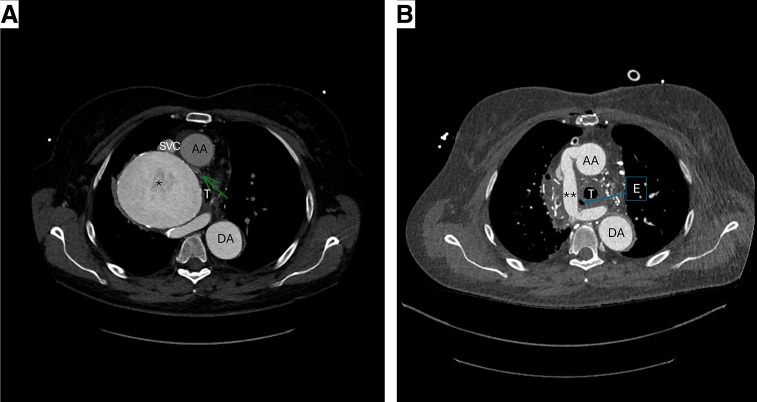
(A) Preoperative CTA showing the giant aneurysm of the aberrant retroesophageal artery (*) connecting the ascending and descending aorta responsible for the brachiocephalic left trunk compression (green arrow). (B) “Postoperative CTA demonstrating the Dacron graft () anastomosed between the ascending and descending aorta. AA: ascending aorta; DA: descending aorta; SVC: superior vena cava; T: trachea; E: esophagus; CTA: computed tomography angiography
